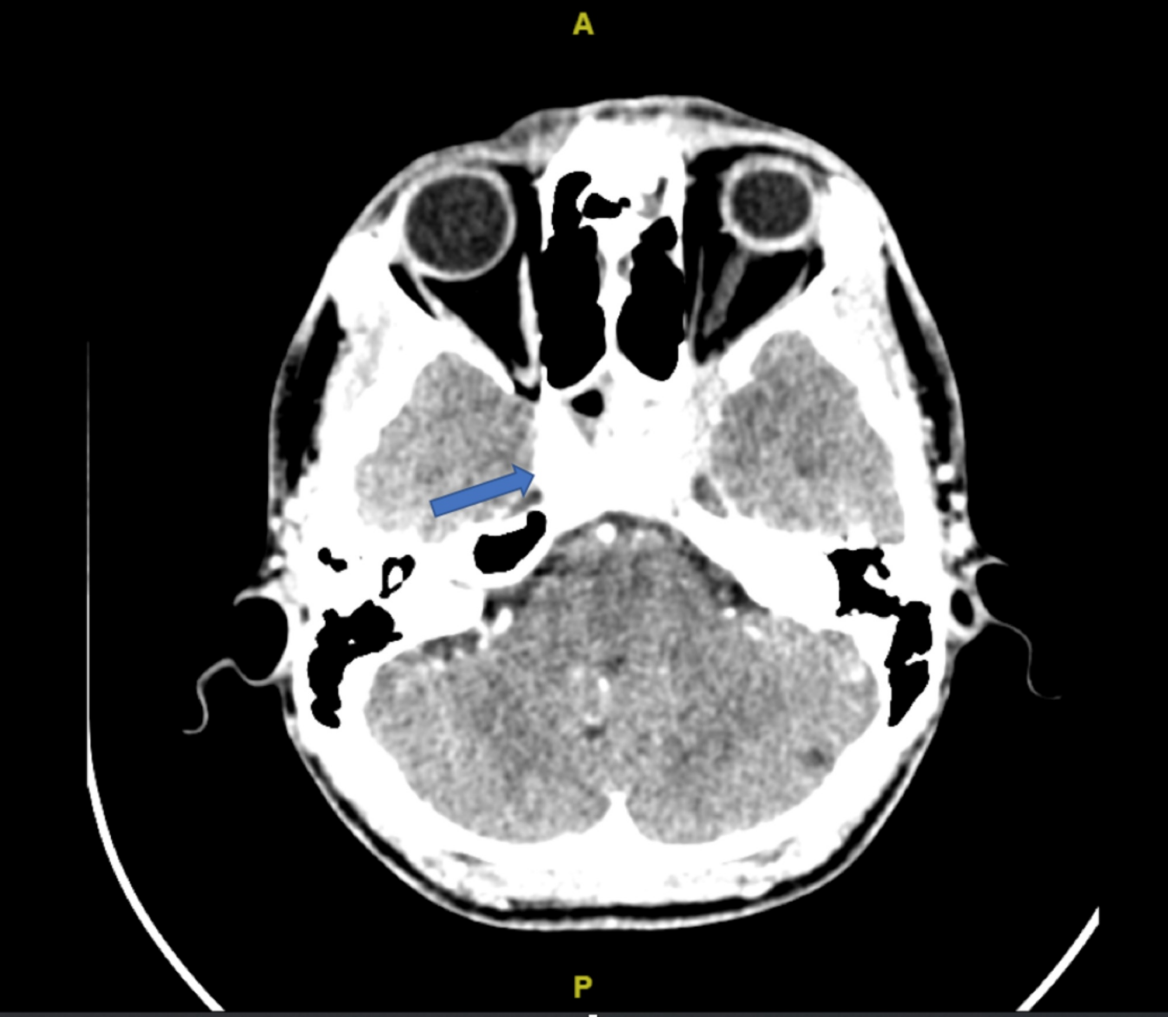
CT scan of the cavernous sinus Arrow pointing towards vascular-enhancing lesions on the right cavernous sinus with filling defects especially at the posterior part. CT: computed tomography
